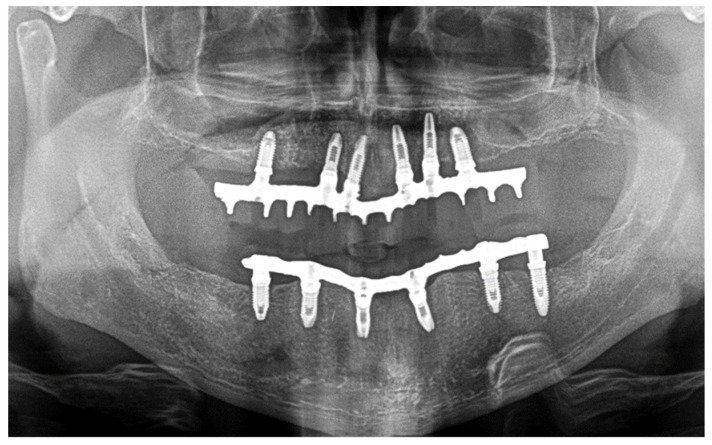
Panoramic radiograph after prosthetic rehabilitation with an implant-fixed prosthesis.
